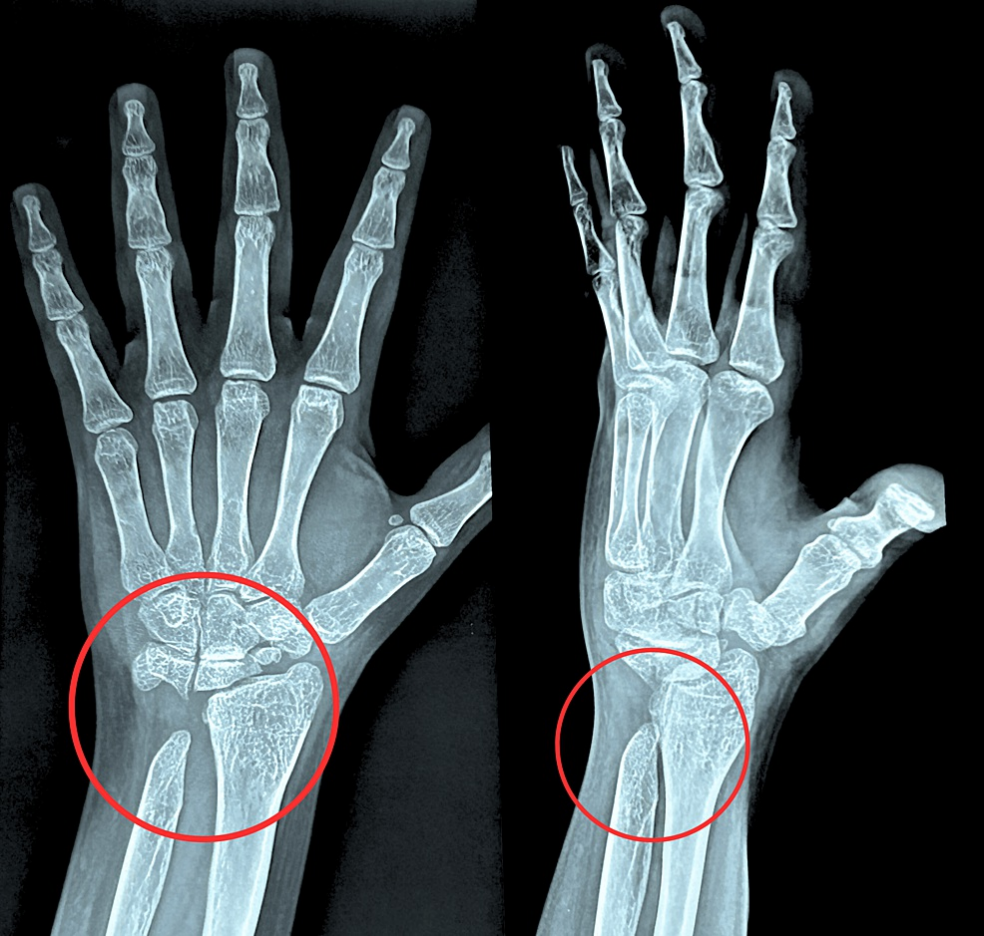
Posteroanterior and lateral views of left wrist radiographic images. The red circle shows an absence of the left ulnar styloid process, indicating distal ulnar hypoplasia as well as fragmented scaphoid.
